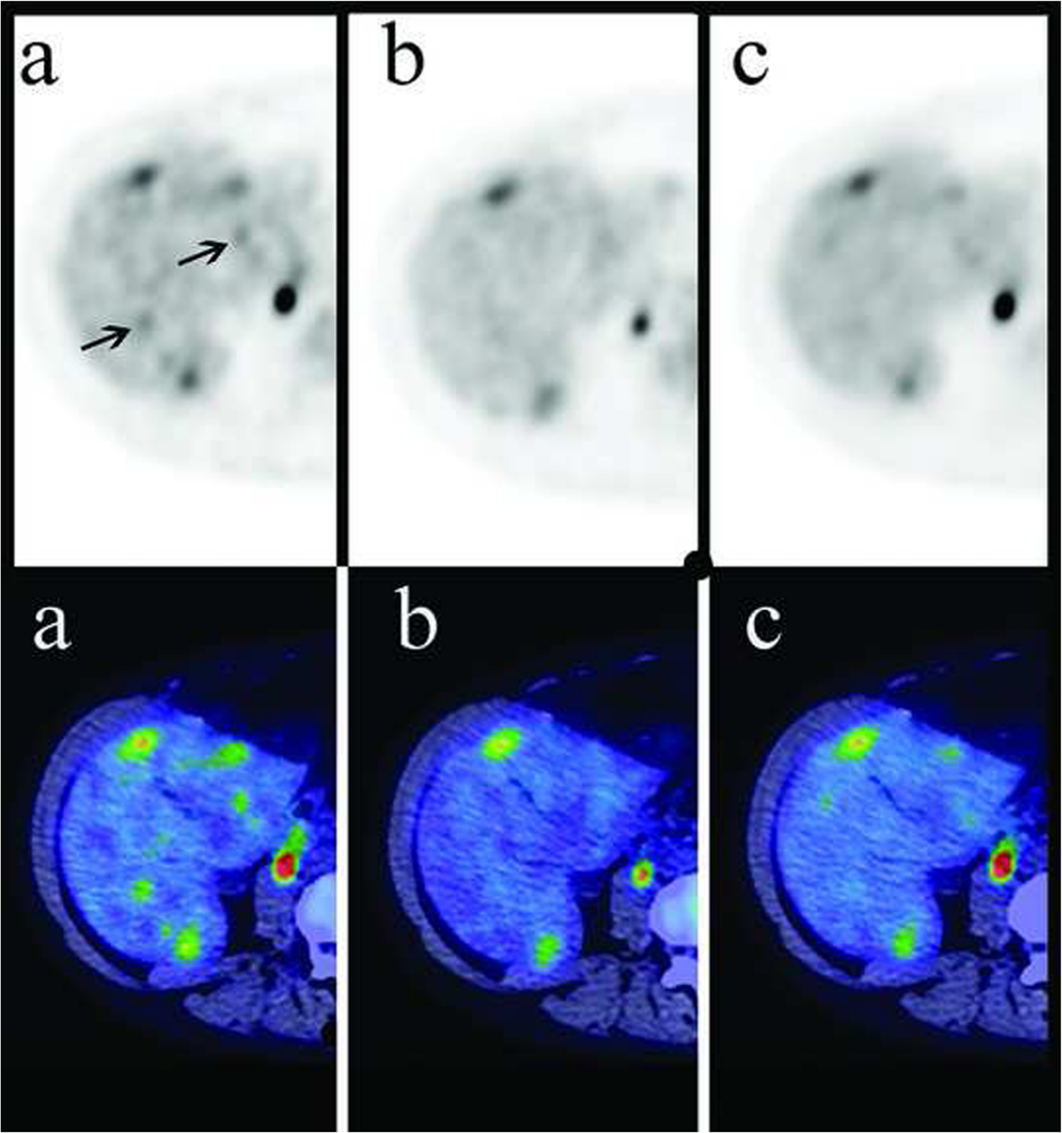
18F-FDG liver biodistribution in a patient affected by liver metastases and referred to PET/CT to restage the disease after systemic therapy. PET and Fusion images were evaluated applying the same SUV_max_ threshold. a Scan with reduced number of counts and standard acquisition (no processed image): highly inhomogeneous radiotracer uptake showing multiple focal uptakes that might be interpreted as metastases and thus, erroneously, as progressive disease. b Native scan (acquisition with increased time): regular distribution of the radiotracer leading to the correct identification of few metastatic lesions (scan correctly reported as stable disease). c Processed scan, obtained with reduced dose and processed by SubtlePET: regular radiotracer distribution in the liver, confirming the non-inferior quality of the image compared to native scan. The interpretation of these image lead to the same conclusion derived by the interpretation of the native scan (stable disease)
